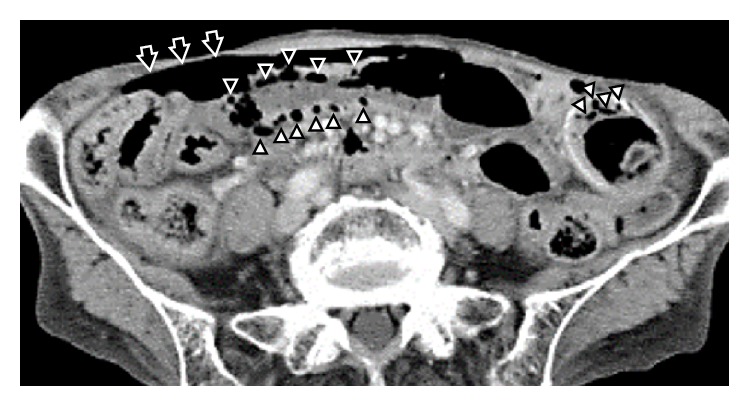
An abdominal computed tomography image demonstrating a large quantity of free air in the abdominal cavity (arrows). Multiple small cysts are seen within the wall of the small bowel (arrowheads), consistent with pneumatosis cystoides intestinalis in this patient with systemic sclerosis.
